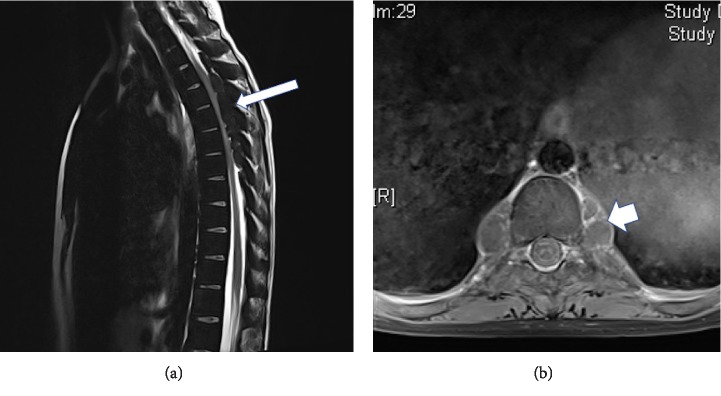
MRI of the dorsal spine: (a) sagittal T2-weighted, (b) axial T1-weighted images with contrast showing posterior epidural multilobulated extramedullary hematopoietic mass from T2 to T9 (arrows) with the largest at T5 causing the most prominent cord compression and showing no contrast enhancement.
